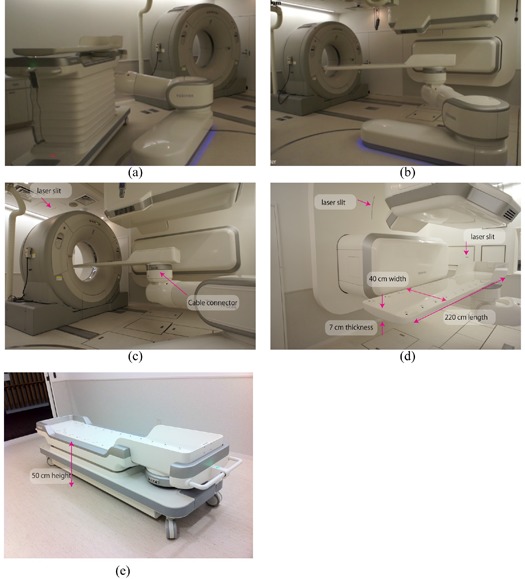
Connection of the robotic arm (a) from the shuttle with the treatment table; (b) transfer of the treatment table to the isocenter by the robotic arm; (c) CT acquisition position; (d) treatment table; (e) shuttle‐down position.
